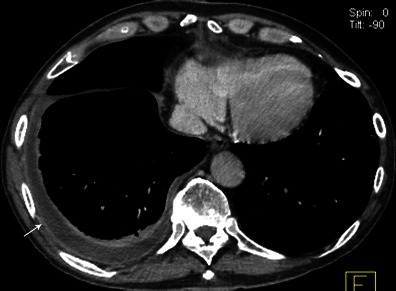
Thoracic CT shows pleural collection with enhancement of pleurae (*white arrow*)
